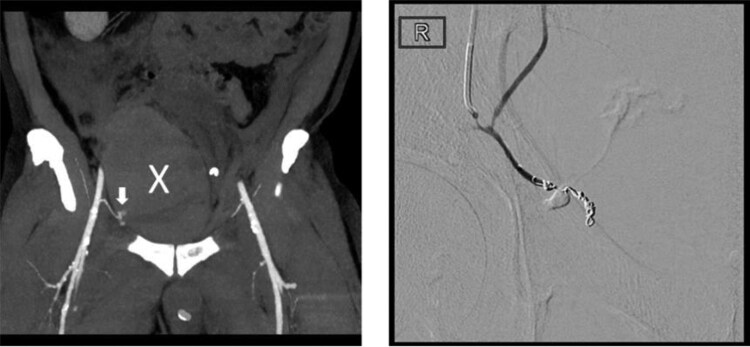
Left: CT scan with contrast showing active bleeding (arrow) and a large retroperitoneal haematoma (X) following vascular access for pulmonary vein isolation in an obese patient (BMI 32 kg/m²). Bleeding source was the right obturator artery which has a variant originated from the external iliac artery. Right: digital subtraction angiography post-embolization (coil). Courtesy of Dr. N. Thieme, Charité Universitätsmedizin, Berlin. BMI, body mass index; CT, computed tomography.
